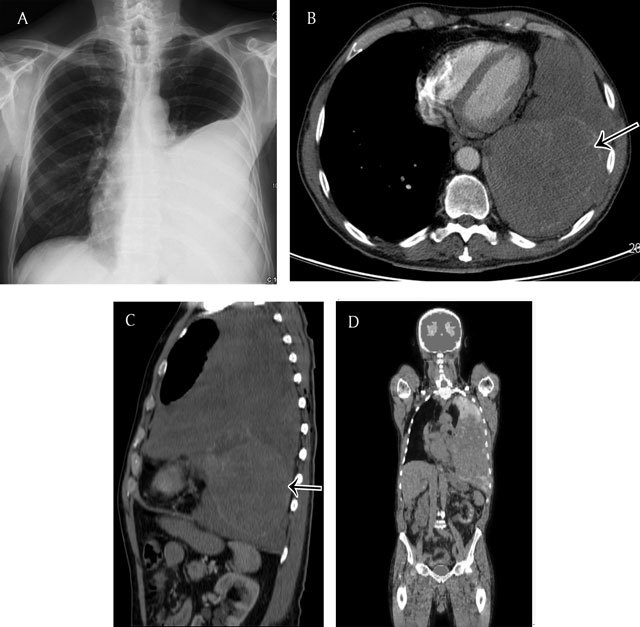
71-year old man with dyspnea and dry cough since 3–4 months. Chest radiograph (a) shows a large left pleural effusion. Axial (b) and sagittal (c) CT image of the chest demonstrates a soft tissue mass occupying the left pleural space. Note the enhancing component at the lower part of the mass (black arrows). Body PET-CT (fluoro-deoxyglucose) (d) shows a hot spot located at the upper part of the pleural tumor. Pathology revealed a giant solitary fibrous tumor (partially hypermetabolic on PET-CT) of the pleura with sarcomatous transformation (enhancing component).
